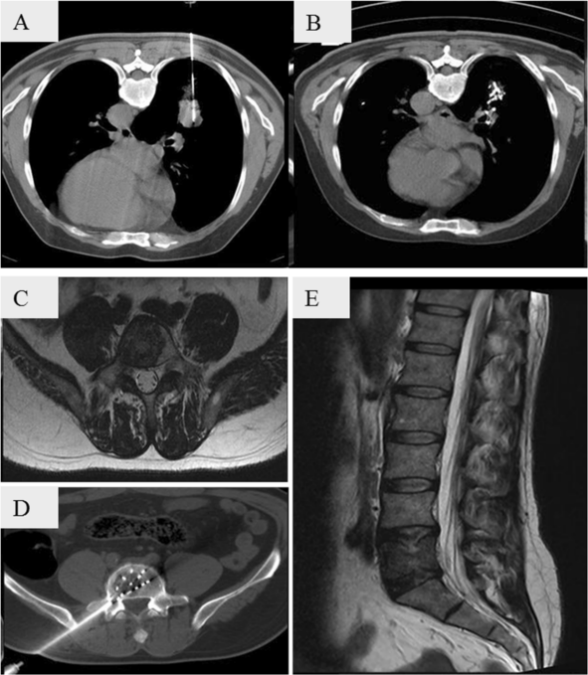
CT images showing brachytherapy of non-small cell lung adenocarcinoma (3 × 2 cm^2^) with vertebral metastasis in a 57-year-old man (No. 17). Both the adenocarcinoma region in the lower right lung (a) and the vertebral metastasis region (c, d) were implanted with iodine-125 seeds. One-year follow-up images showed good tumor control in both regions (b, e)
